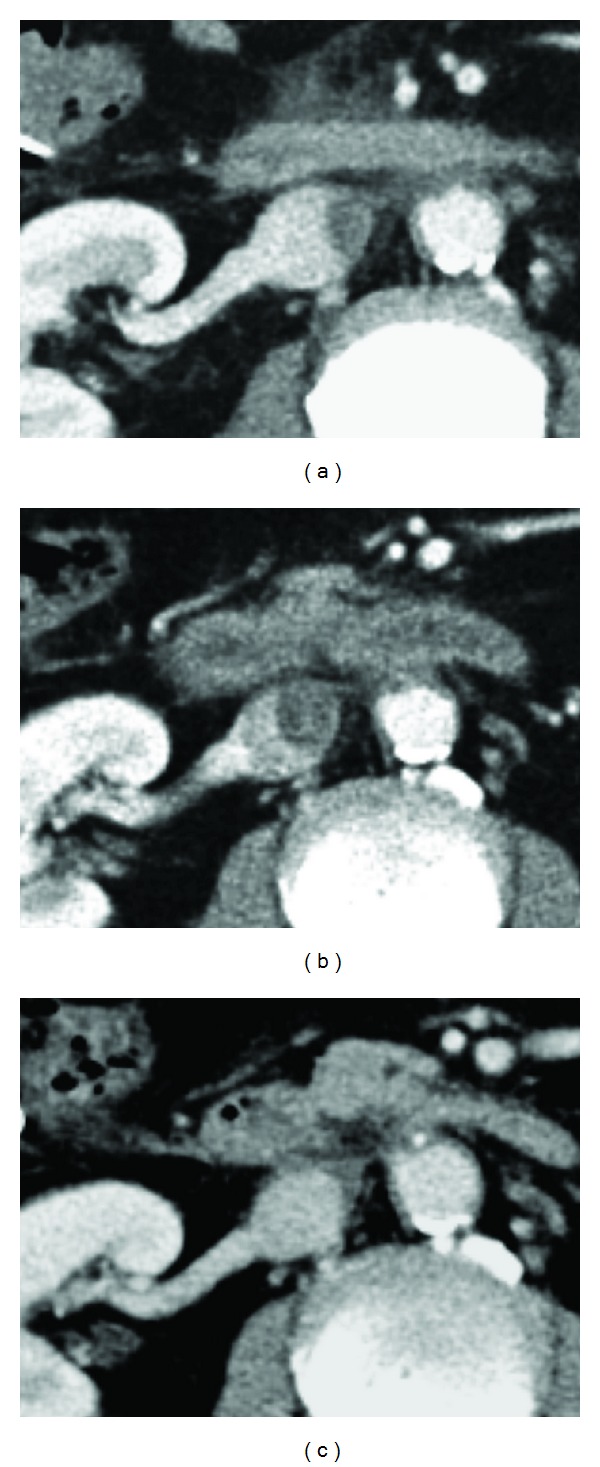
Contrast-enhanced abdominal computed tomography. (a) Mural thrombus extensively adhered to the vessel wall in the infrarenal inferior vena cava (IVC). (b) Anticoagulant therapy with unfractionated heparin and antithrombin was administered, but the IVC thrombus continued to increase in size, five days later. (c) After switching to warfarin and fondaparinux sodium anticoagulant therapy, the IVC thrombosis significantly shrank within one month.
